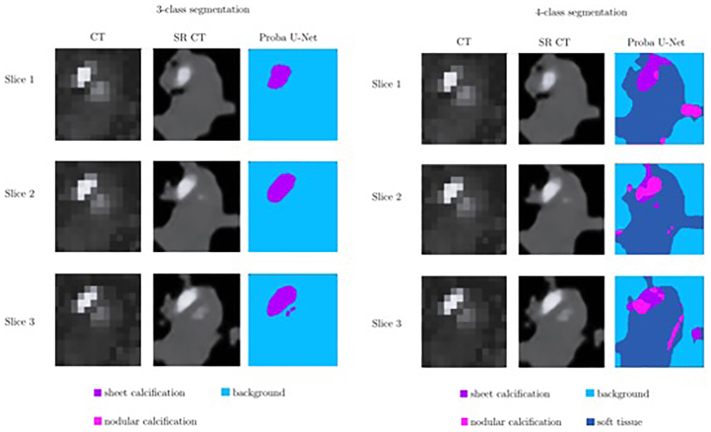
The three-class model: Three consecutive super-resolution (SR) (×8 LapSRN) computed tomography (CT) slices and their segmentations produced by the Probabilistic U-Net model, under three-class (such as background, nodular calcification, and sheet calcification). The consecutive nature of the slices demonstrates the spatial consistency of the segmentation: the predicted regions evolve smoothly across slices, confirming that the model captures meaningful anatomical structure rather than producing random outputs. The four-class model: Three consecutive SR-CT slices and their segmentations produced by the Probabilistic U-Net in the four-class configuration (such as background, soft tissue, nodular calcification, and sheet calcification). Adding the soft-tissue label highlights the network’s capacity to distinguish noncalcified plaque; the smooth evolution of all four regions across slices attests to spatial consistency.
